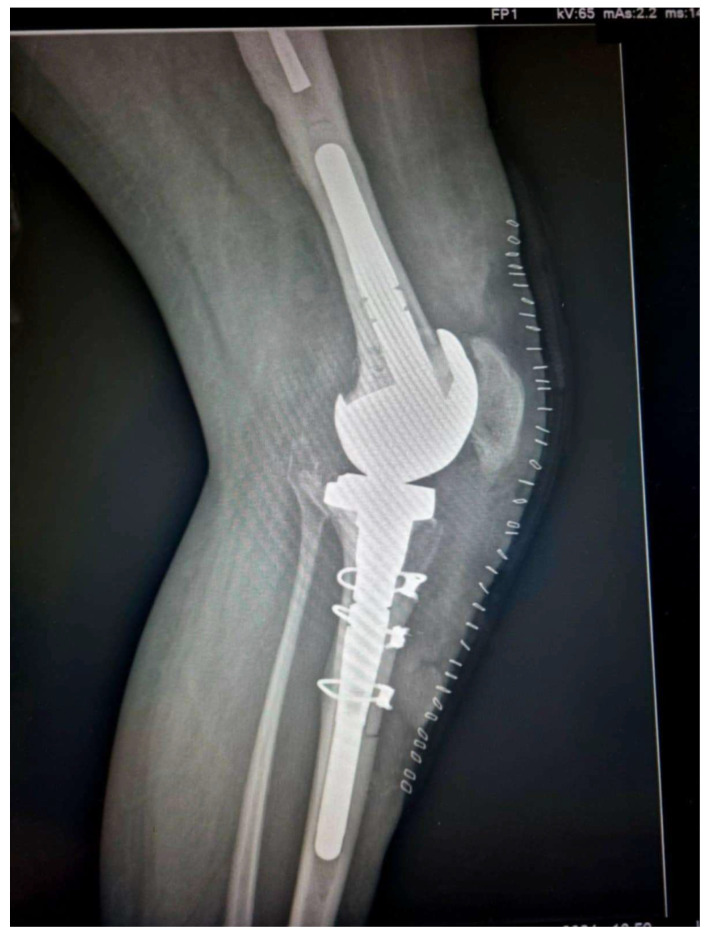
Postoperative radiograph demonstrating the proper reduction of the bone flap (Case 3).
